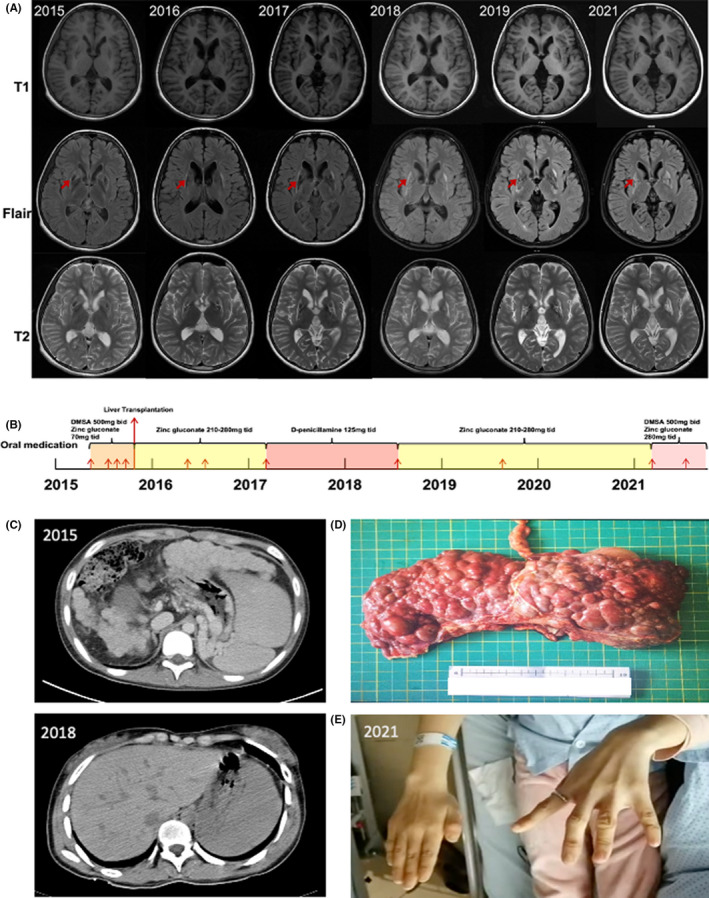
(A) Cranial MRI of the patient from 2015 to 2021, with metal deposition visible in the region of the lenticular nucleus (shown by red arrows). (B) Timeline of the patient's treatment process. Small red arrows stand for single intravenous dose of sodium dimercapto sulfonate (DMPS) copper chelation therapy that the patient received at each hospitalization. DMSA stands for dimercapto succinic acid. (C) CT scan images of the patient's liver in 2015 before LT and in 2018. (D) Intraoperative patient's liver pathology. (E) Patient's left‐hand dystonia manifested in 2021.
